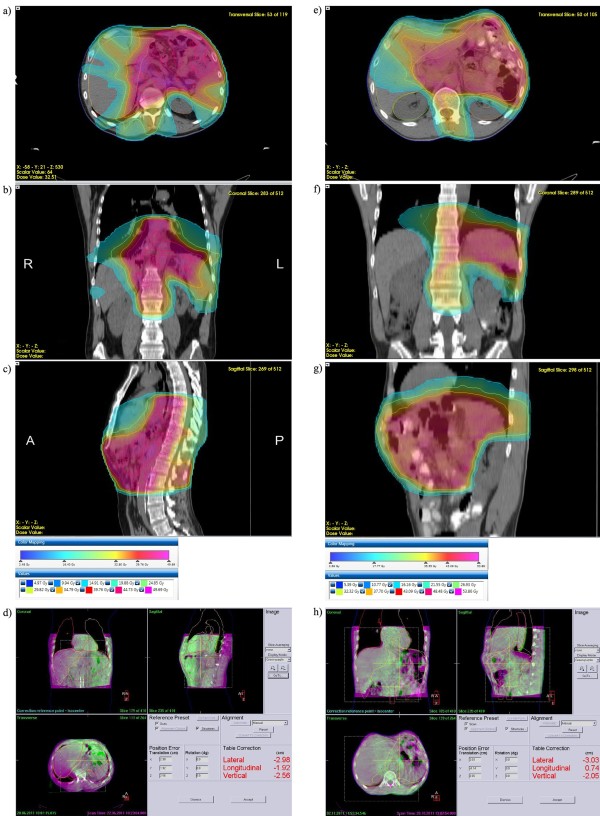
VMAT treatment plans for gastric and GEJ carcinomas. (a-c): Volumetric intensity-modulated arc therapy (VMAT) plan (Monaco®, Elekta, Crawley, UK) of a patient with a junctional carcinoma in the adjuvant situation. Transversal (a), coronal (b), and sagittal (c) dose distributions. Color wash and isodose lines are indexed in the insert. A total dose of 45Gy was applied. Note the expansion of the planning target volume (PTV) to the distal esophagus and sparing of the posterior wall of the heart, both lungs, and the dorsolateral renal cortex in the left kidney. (d): Cone beam computed tomography (CBCT) based position correction directly before the first therapy fraction after positioning based on skin marks. Magenta: planning computed tomography (PCT); green: CBCT; both PCT and CBCT in mid-ventilation position. Manual position correction was based on internal surrogate structures such as calcifications in the aorta. (e-g): VMAT plan of a patient with stomach (corpus) carcinoma in the adjuvant situation. Transversal (e), coronal (f) and sagittal (g) dose distributions. Color wash and isodose lines are indexed in the insert. A total dose of 45Gy was applied. Note the sparing of the dorsolateral renal cortex in the left kidney and the complete right kidney. (h): CBCT based position correction directly before the first therapy fraction. Magenta: PCT; green: CBCT.
